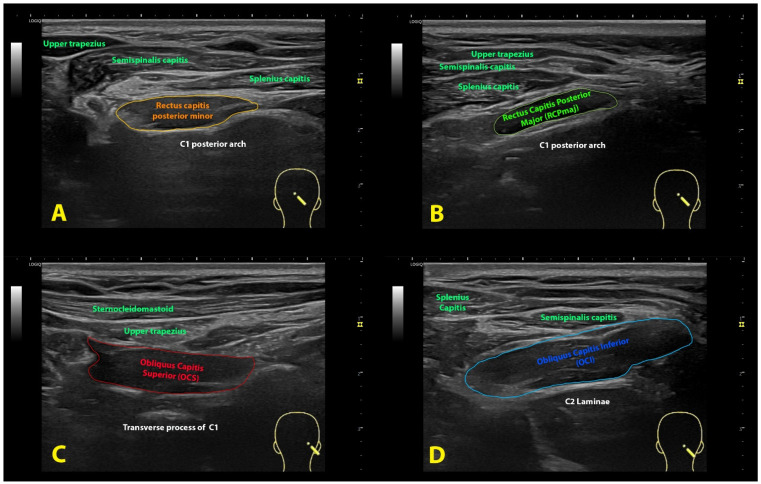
Ultrasound imaging of the rectus capitis posterior minor (A), rectus capitis posterior major (B), oblique capitis superior (C) and oblique capitis inferior (D).
